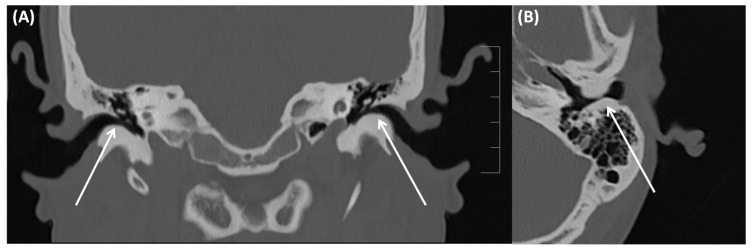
CT of a 48-year-old male patient with a long history of surfing and sailing. The patient was mostly asymptomatic, reporting problems with water getting into the ear that drains poorly. Coronal image (A), and axial image (B) using bone windowing.Exostosis of the EAC on both sides can be identified (arrows).
